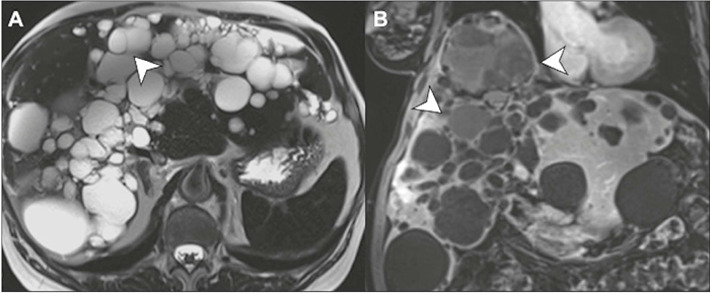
Polycystic liver disease on MRI. Note that, as with renal
